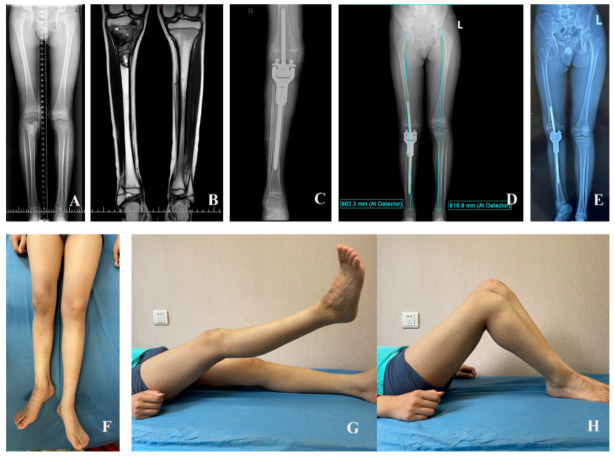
The preoperative X-ray film shows a 10-year-old boy (patient No. 1) with osteosarcoma of the right proximal tibia (A); postoperative MRI (B); postoperative X-ray film (C); the full-length standing anteroposterior radiographs were taken 12 months (D) and 45 months (E) after the initial surgery; the range of motion of the knee after rehabilitation was satisfied (F–H).
